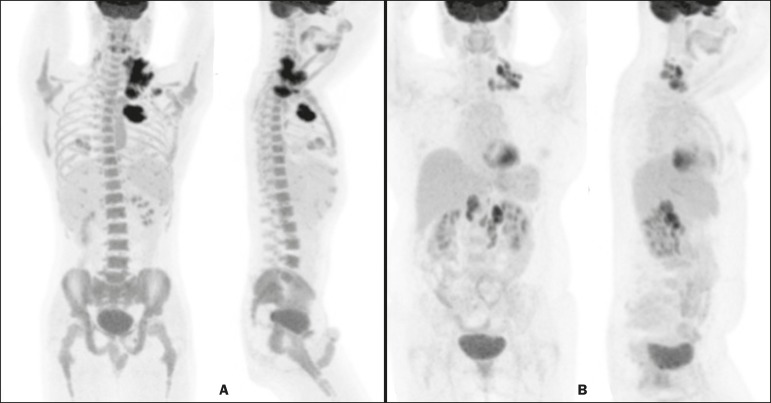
^18^F-FDG PET/CT studies of two patients-one with B symptoms
